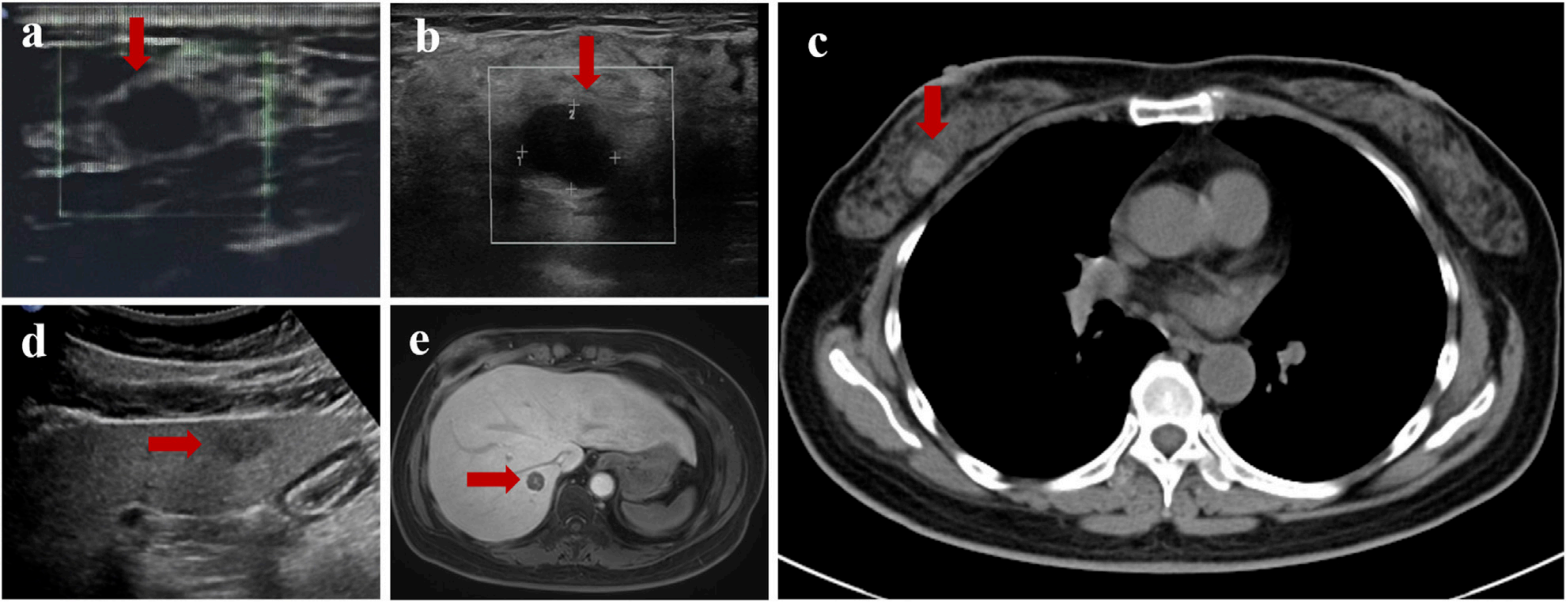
Imaging findings of this case. (a) Ultrasonography revealed a low-density mass measuring 1.1 cm* 0.8 cm in the right breast in 2016. (b) Ultrasonography revealed a 1.3 cm*1.0 cm mixed echogenic mass with clear boundaries in the right breast in 2024. (c) Computed tomography (CT) showed a high-density mass measuring 1.4 cm in the right breast in 2024. (d) The largest hypoechoic mass the abdominal ultrasound showed in the liver in 2024, measuring 1.6*1.4 cm in size. (e) The largest round-shaped abnormal signals displayed by magnetic resonance imaging (MRI), with a diameter of 1.8 cm.
